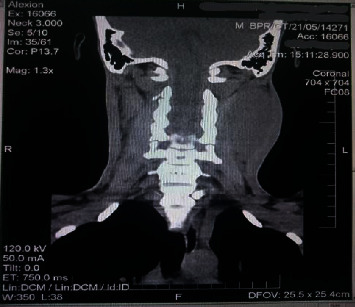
Coronal view of CT neck showing an isodense mass with well-defined edges on the left.
